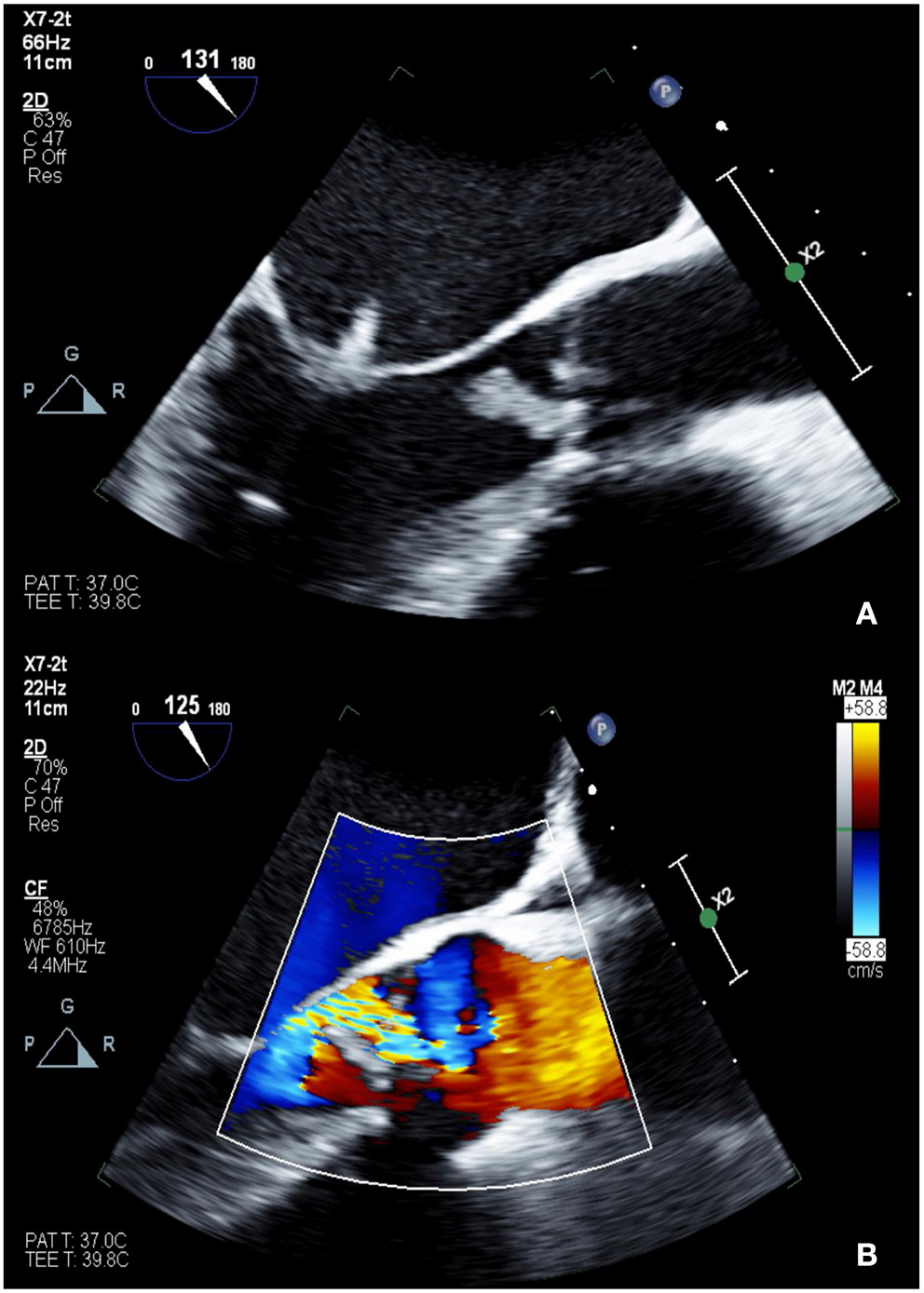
TEE showing 2D (A: top) and color Doppler (B: bottom) images of Libman-Sacks endocarditis involving the left-sided valves (A) and severe eccentric aortic insufficiency (B). The patient was found to have positive lupus anticoagulant as well as high titers of anti-cardiolipin IgM and anti-beta-2 glycoprotein I IgM, consistent with triple positive APLS.
